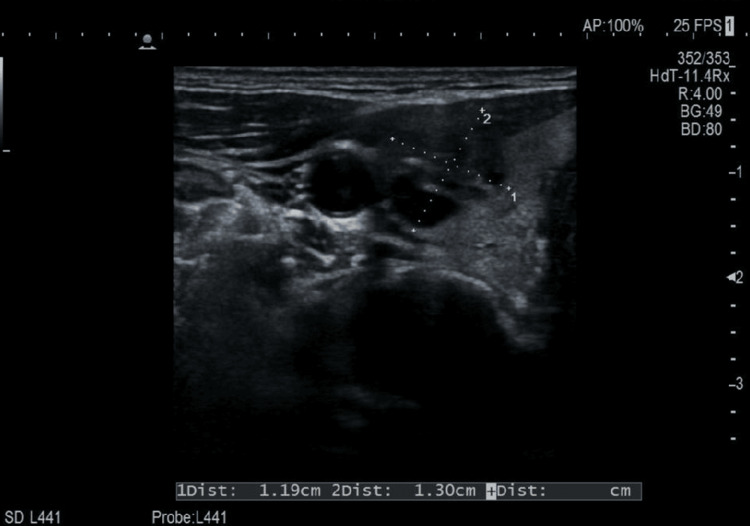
Cervical lymph node ultrasound Transverse ultrasound image of a left-sided cervical lymph node (level IV) demonstrating a hypoechoic cortex and central echogenic hilum. Dimensions: 1.19 × 1.30 cm.
